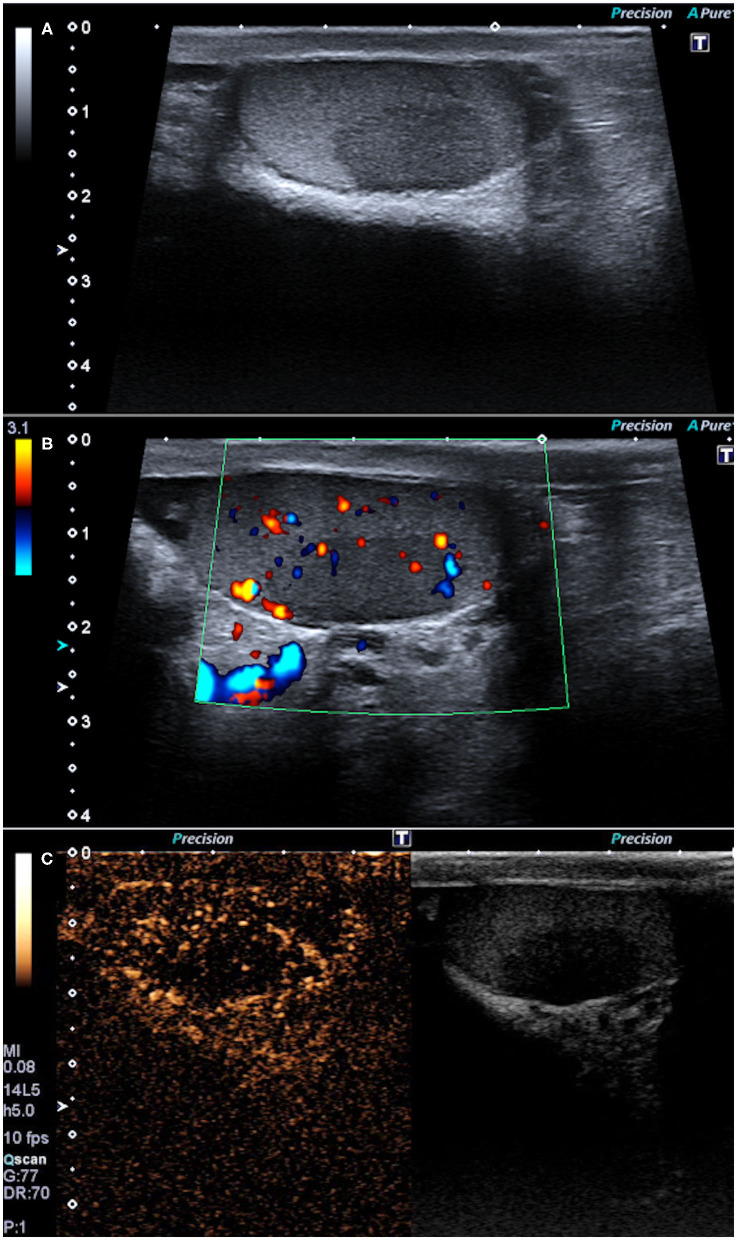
B-mode ultrasonography showing one hypoechoic lesion in the left testis with ill-defined margins (A) and some Color-Doppler flow (B). Contrast-enhanced ultrasonography showing the hypovascular appearance of the lesions as compared to the surrounding testicular tissue (C).
